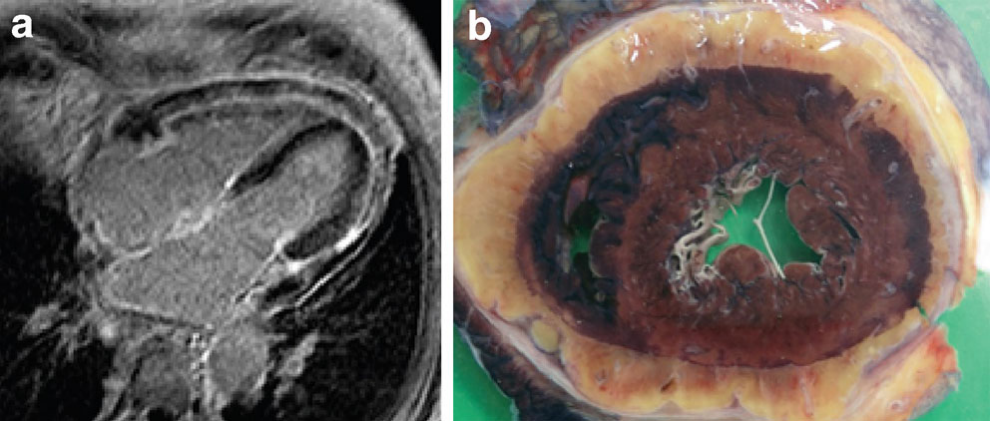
a Patchy enhancing pericardial soft-tissue on late-gadolinium enhanced MRI, b Macroscopic image of the heart with pericardial amyloid deposition
